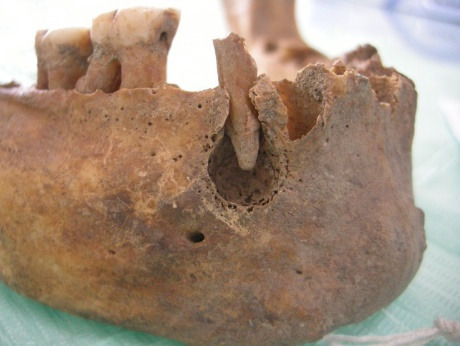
Caries de localización coronaria y afectación pulpar. Obsérvese la amplia lesión periapical con destrucción del hueso.
